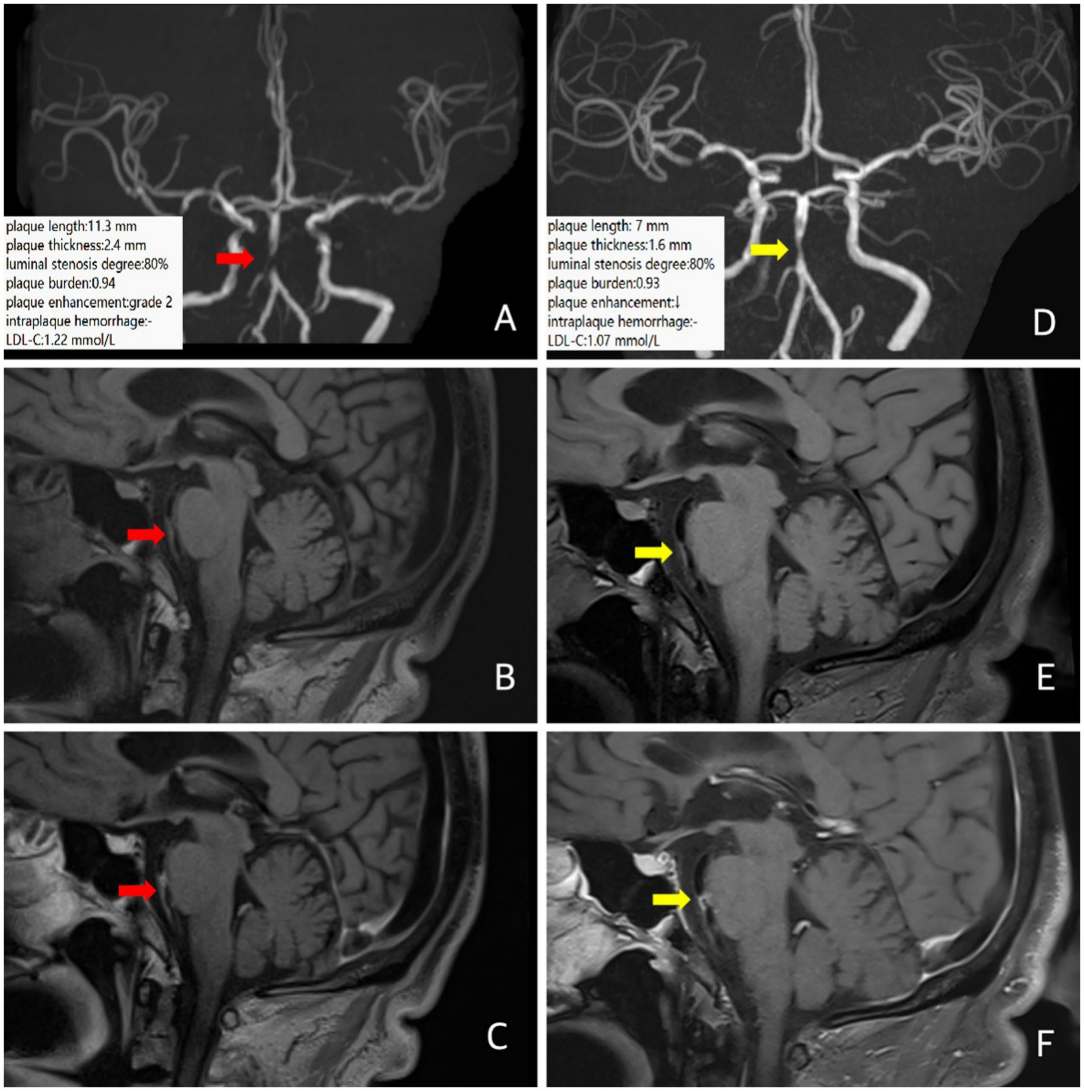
In case 1, patient at baseline (red arrow) and follow-up images 12 months later (yellow arrow). Three-dimensional time-of-flight cerebral magnetic resonance angiography showed significant stenosis in the middle segment of the basilar artery (A,D). Coronal T1 plain scan weighted imaging (B,E) and enhanced imaging (C,F) in whole brain magnetic resonance imaging using different inversion angles to optimize contrast showed multiple atherosclerotic plaque formation in the basilar artery with obvious stenosis in the middle lumen and obvious plaque enhancement. Follow-up intracranial VW-MRI showed that compared with baseline, the length of the plaques was shortened, the plaque thickness was attenuated and there was no significant change in luminal stenosis, plaque burden, or plaque enhancement. Still, the overall enhancement segment was shortened, suggesting that the plaque tended to be stable. The LDL-C levels decreased.
